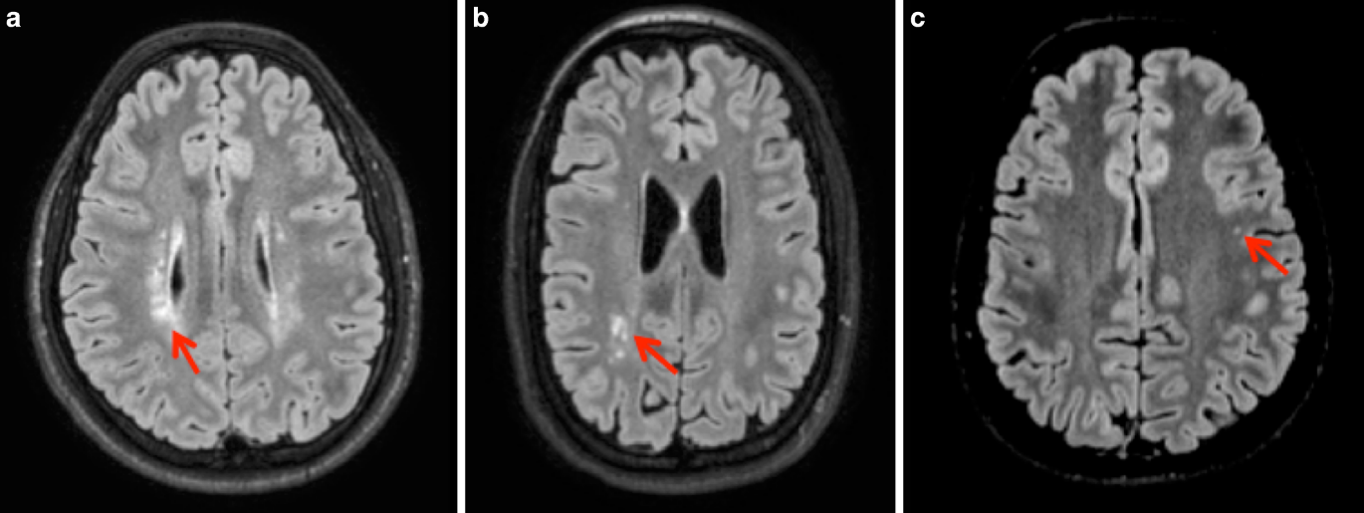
Fluid-attenuated inversion recovery (FLAIR) lesion localization. Examples of FLAIR hyperintense white matter lesions in periventricular (a), deep white matter (b) or subcortical (c) localization. Periventricular FLAIR lesions abut the ventricular surface, subcortical FLAIR lesions lie within 3 mm of the cortical ribbon, without direct contact. Representative lesions are marked with a *red*
